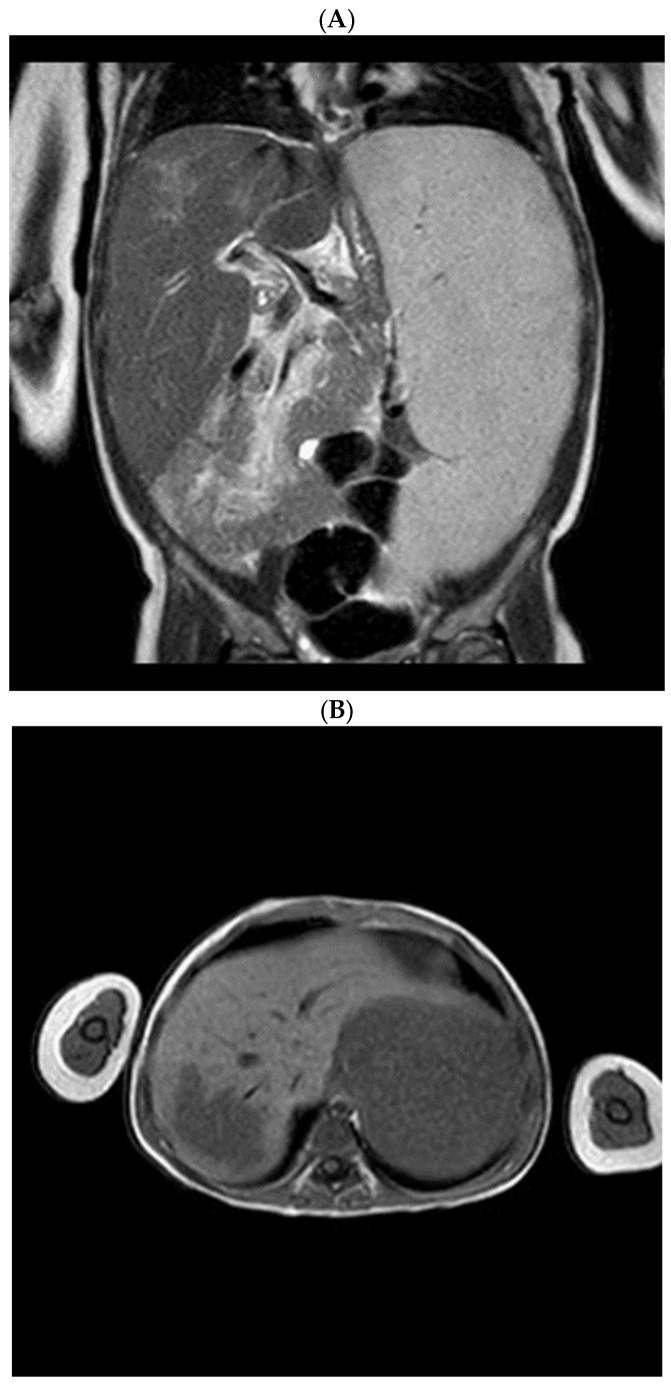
Magnetic resonance imaging shows massive splenomegaly and hepatomegaly (A), with a focal hepatic lesion characterized as gaucheroma (B).
